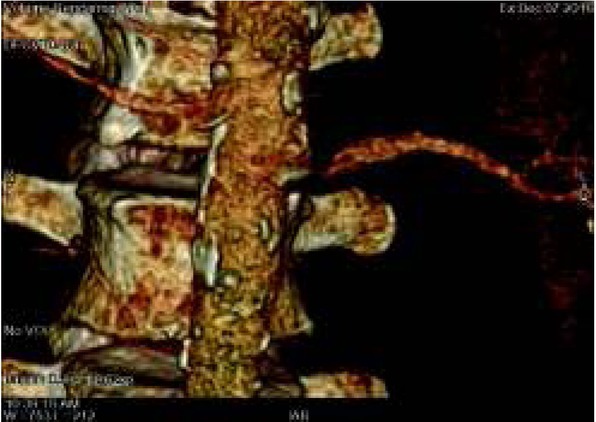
CT Angiography. Left renal artery stenosis. Ryc. 5. Angio CT. Zwężenie lewej tętnicy nerkowej w miejscu odejścia od aorty.
